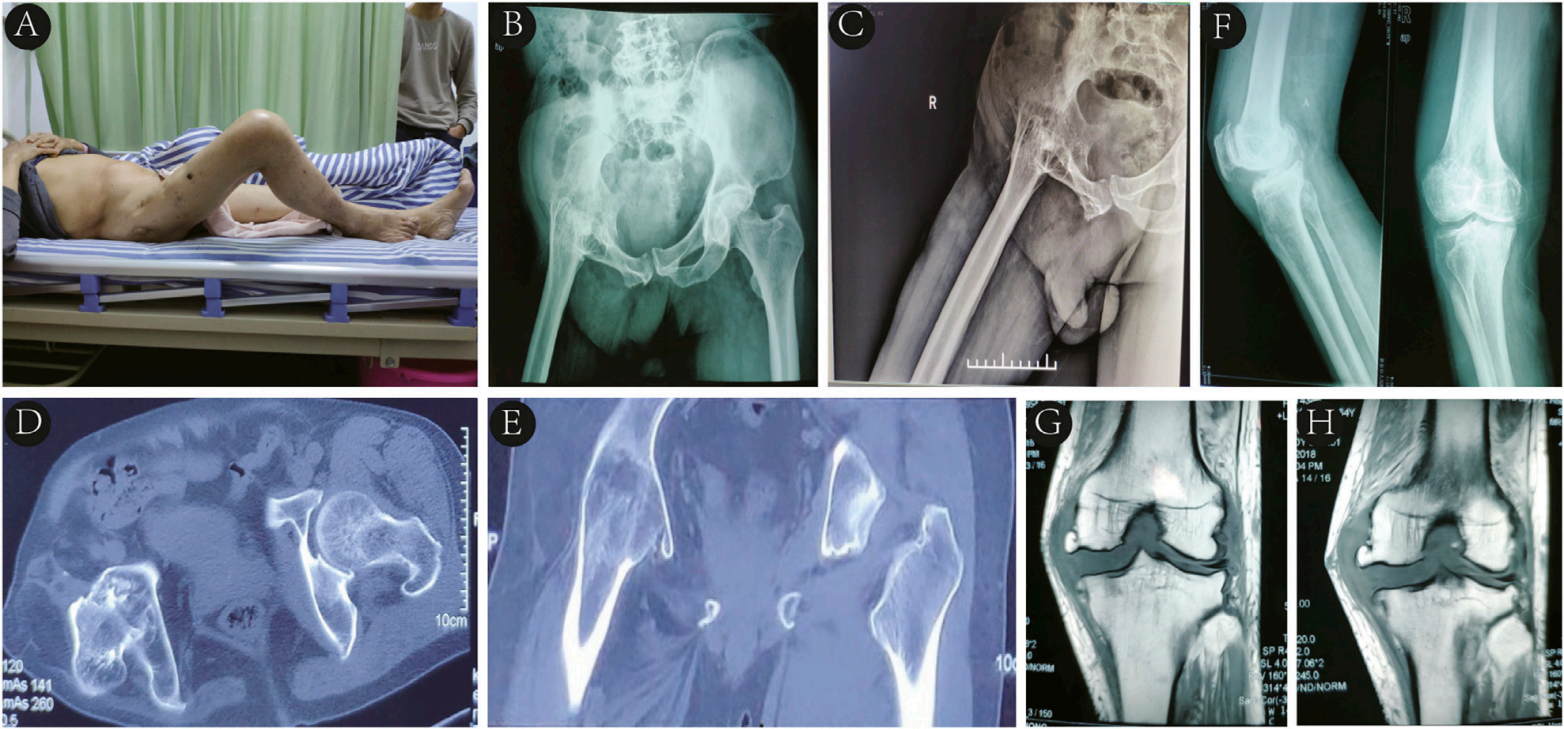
(A) Right hip joint fixed in 45° of flexion and 30° of abduction and external rotation. (B–E) Pelvic X-rays in standard position, lateral hip X-rays, and CT imaging revealing pelvic tilt, right hip ectopic fusion, and femoral neck near-total disappearance. (F, G, H) Right knee X-rays and MRI displaying degenerative changes, distal femoral and proximal tibial osteophyte formation, and lateral collateral ligament injury.
